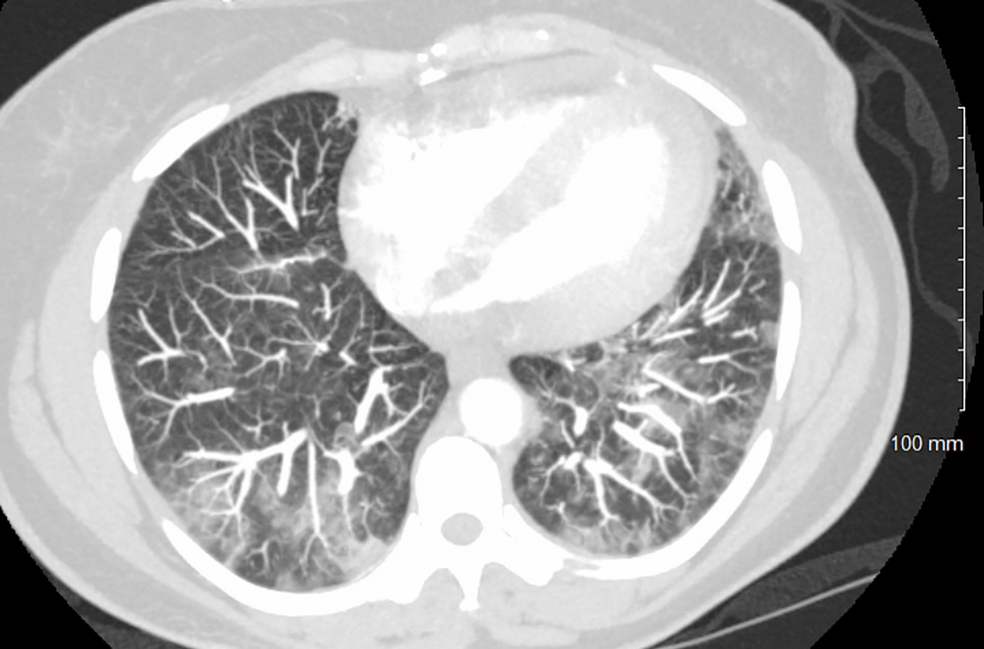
CT angiogram was negative for pulmonary embolism, however, did show multifocal airspace opacities consistent with COVID-19, small to moderate sized pericardial effusion.
